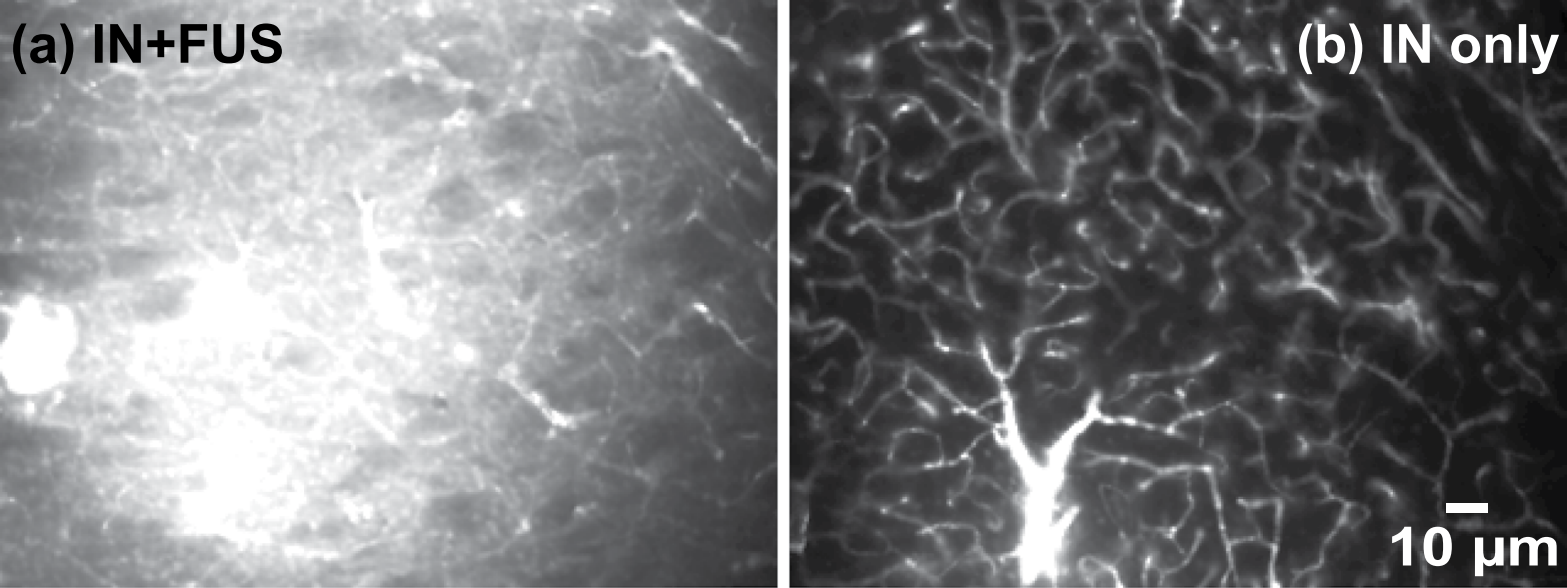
Higher magnification images present the locally-enhanced delivery effect of FUS on IN drug delivery. (a) FUS sonication enhanced dextran penetration and diffusion at the focused ultrasound targeted region. (b) Without FUS sonication, the fluorescently-labeled dextrans were confined within the perivascular space.
